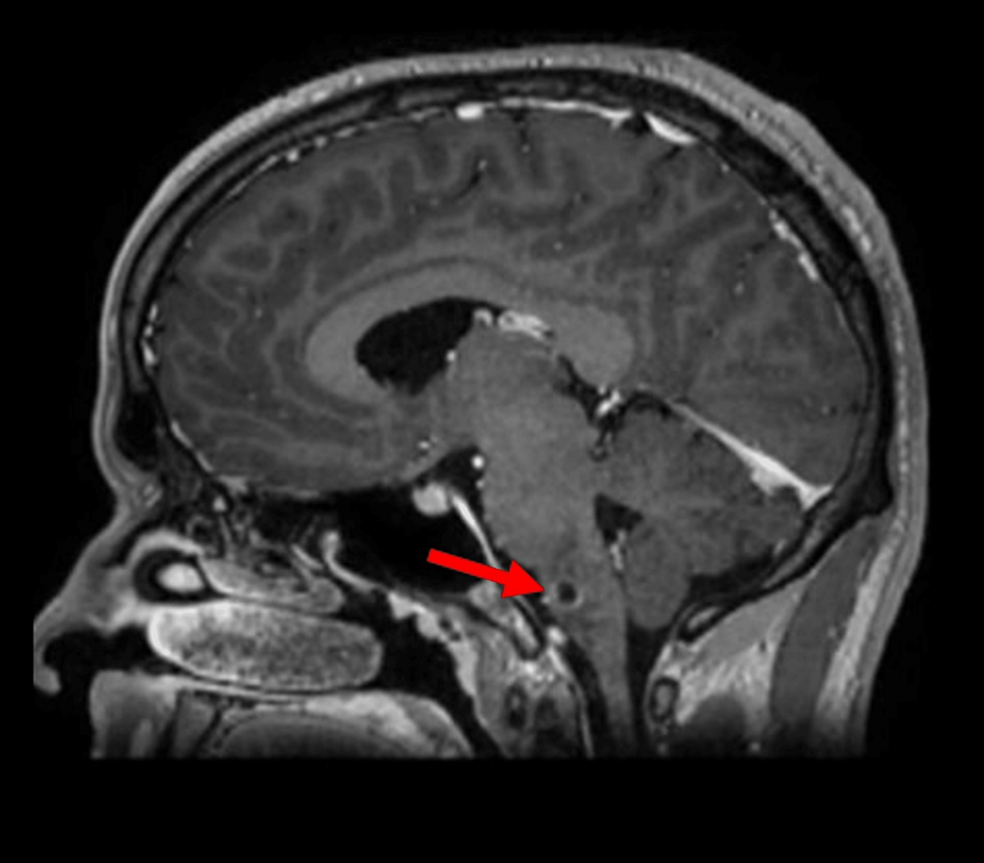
Sagittal T1-weighted contrast-enhanced brain MRI demonstrating a hypointense ring-enhancing lesion at the left medulla oblongata (arrow).​
